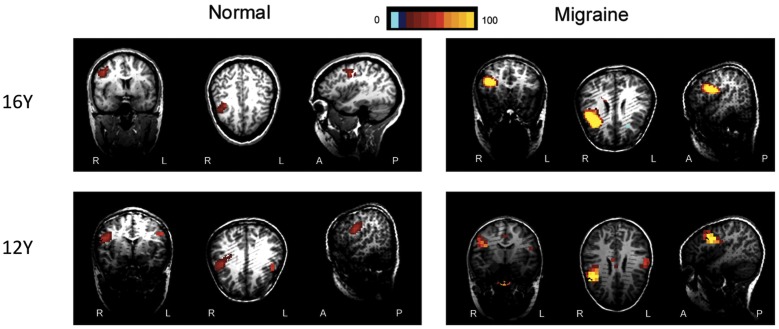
Magnetic source activation of high-gamma oscillations elicited by left finger movement. Magnetic Source Imaging (MSI), the combination of magnetoencephalography (MEG) results and magnetic resonance imaging (MRI), shows the source activation elicited by left finger movement in both children with migraine and healthy controls. The red and yellow areas indicate regions of neuromagnetic activation (or synchronized neural firing). The neuromagnetic activation elicited by left finger movement is localized in the contralateral motor cortex in healthy controls (“Normal”). The neuromagnetic activation elicited by left finger movement is localized in the contralateral motor cortex as well as the premotor (“16 Y”) and supplementary (“12 Y”) motor areas in children with migraine (“Migraine”).
